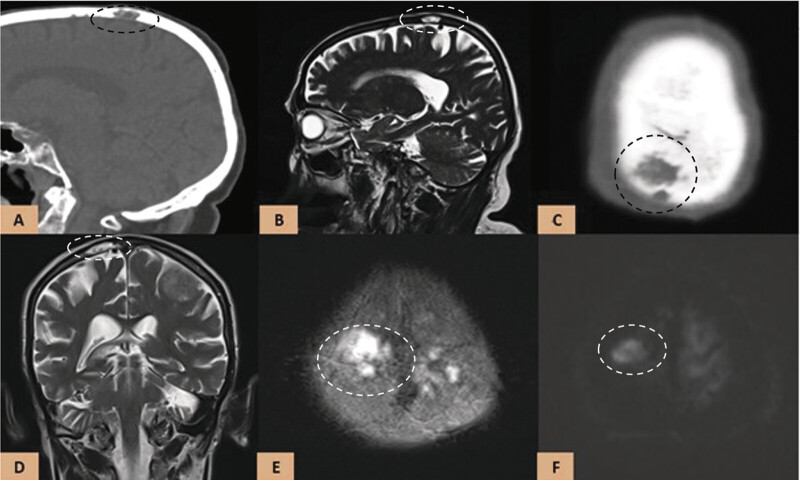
Right parasagittal epidermoid cyst. A cystic hypodense mass lesion (18 × 9 × 13 mm) compressing the superior sagittal sinus sagittal CT image (
